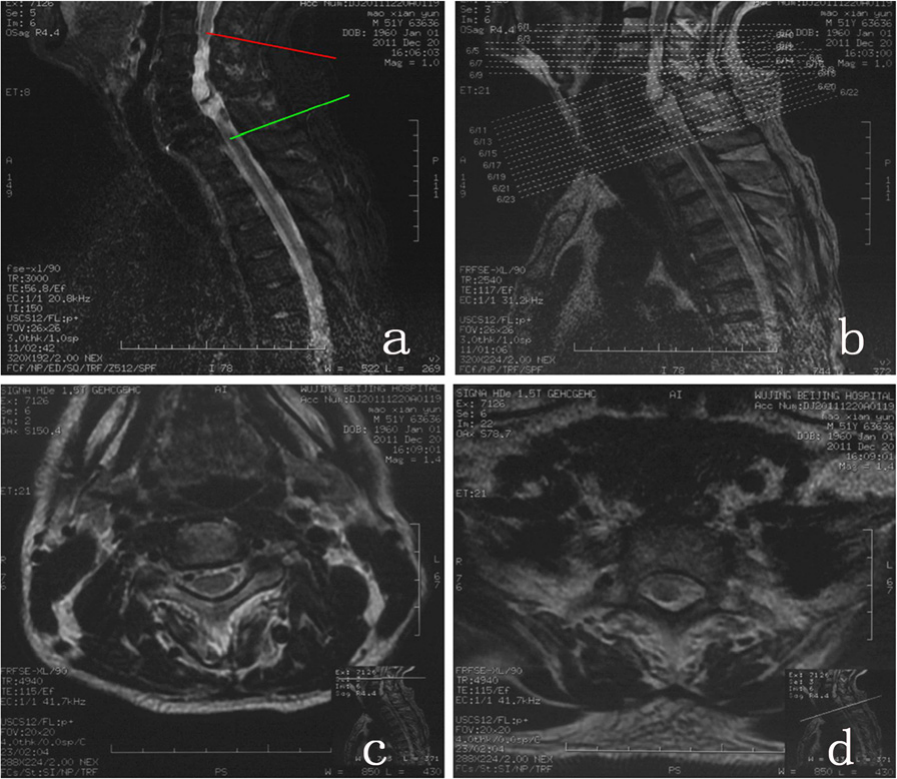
Magnetic resonance imaging ascertainment of the local stem cell transplantation target by CT-guided puncture before operation. a, Cervical MRI indicating that the patient had a C3-C6 injury; thus, the upper intervertebral space to be punctured was C3-C4, and the lower intervertebral space was C7-T1. The red line indicates the upper intervertebral space (C3-C4) to be punctured and the intended target for cell transplantation. The green line represents the upper intervertebral space (C7-T1) for the intended puncture and the intended cell transplantation site. b, c, d, The spinal cord conditions of the upper and lower targets designated for stem cell transplantation, indicating that the targeted sites in the spinal cord were healthy in appearance, contained no obvious lesions and were close to the injury site.
